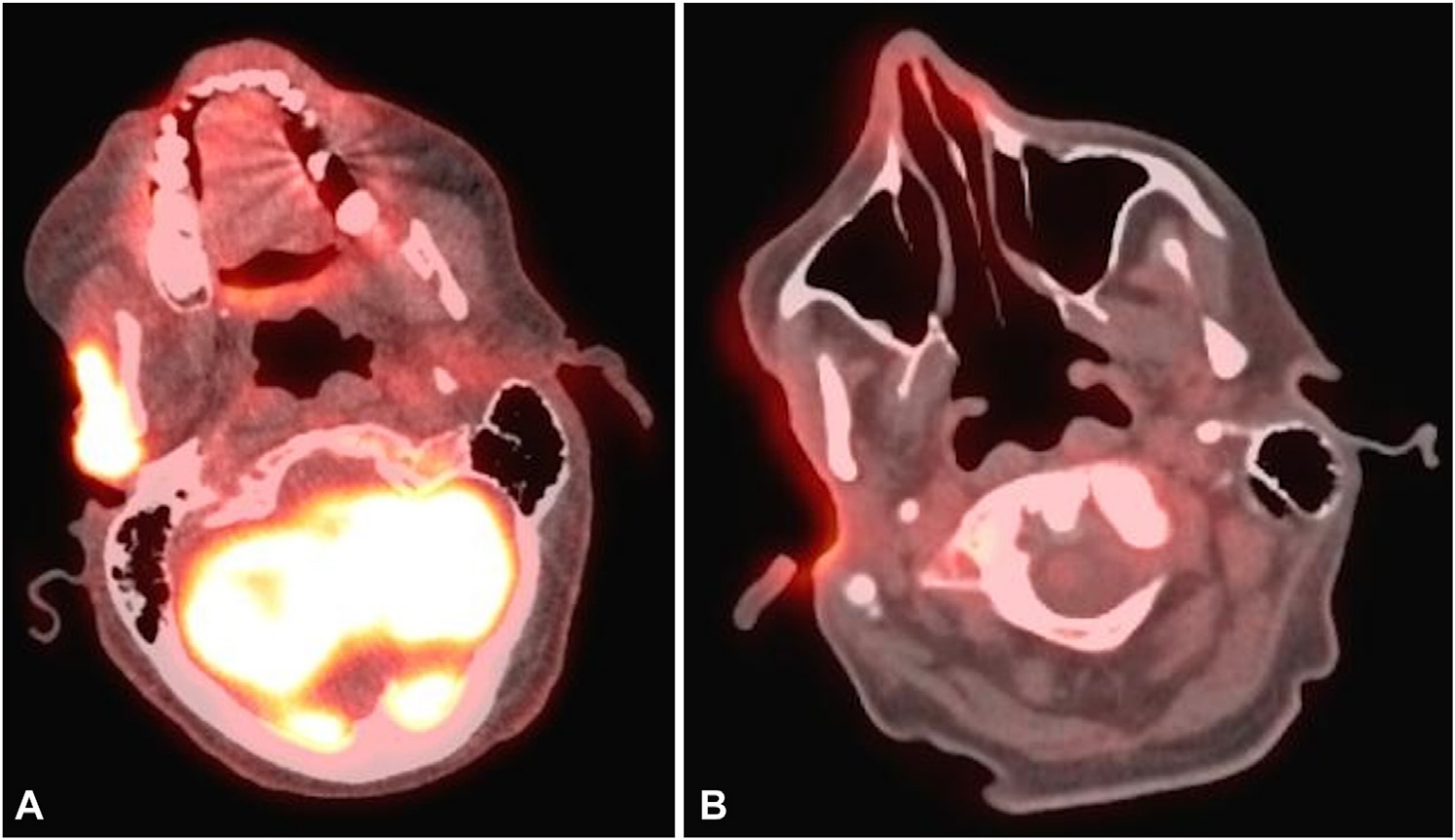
A, PET-CT of head before ruxolitinib taper and starting pembrolizumab treatment. B, PET-CT of head 2 months after ruxolitinib was stopped and after 4 doses of pembrolizumab. *PET-CT*, Positron emission tomography-computed tomography.
